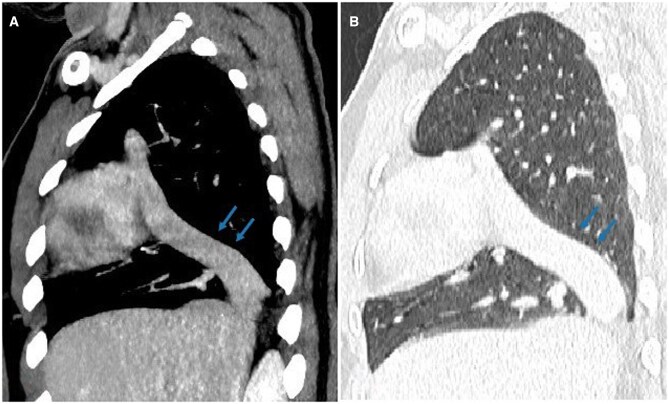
(A) mediastinal window, (B) lung window. The sagittal CT image shows the inferior vena cava crossing the right lung and draining into the right atrium (arrows).
